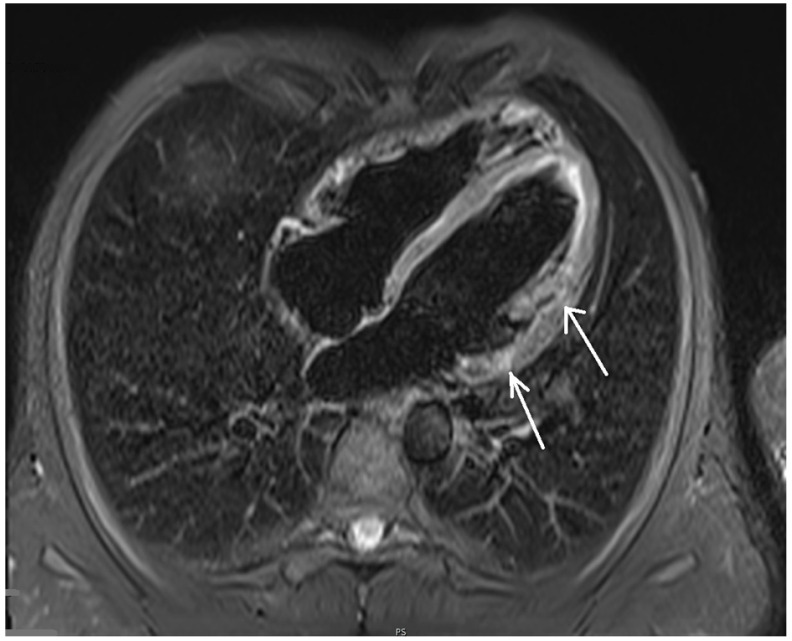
Magnetic resonance imaging of the heart showing disseminated subepicardial and midwall late enhancement lesions here in the lateral wall of the left ventricle (arrows).
